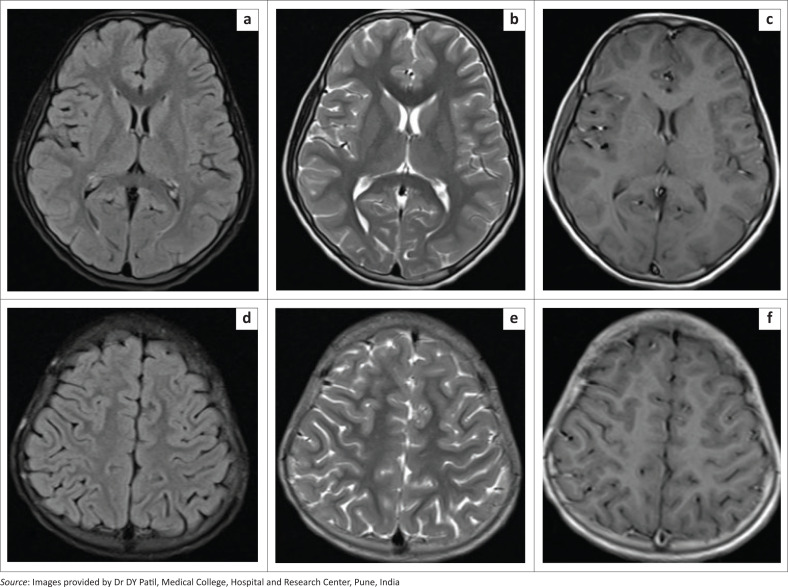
[Case 5]: Repeat brain MRI of a dengue seropositive 10-year-old female diagnosed with posterior reversible encephalopathy syndrome after 4 months showing complete resolution of the symmetrical signal abnormalities in the bilateral temporo-occipital and frontoparietal regions on axial FLAIR (a, d), T2-weighted (b, e) and T1-weighted (c, f) images.
